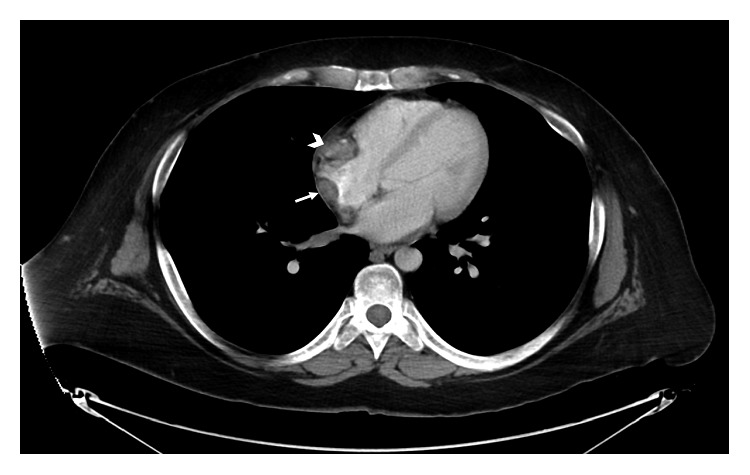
Axial contrast-enhanced CT image of the chest shows nodular soft tissue thickening overlying the right atrium posteriorly (arrow) and extending to the atrioventricular groove (arrowhead).
